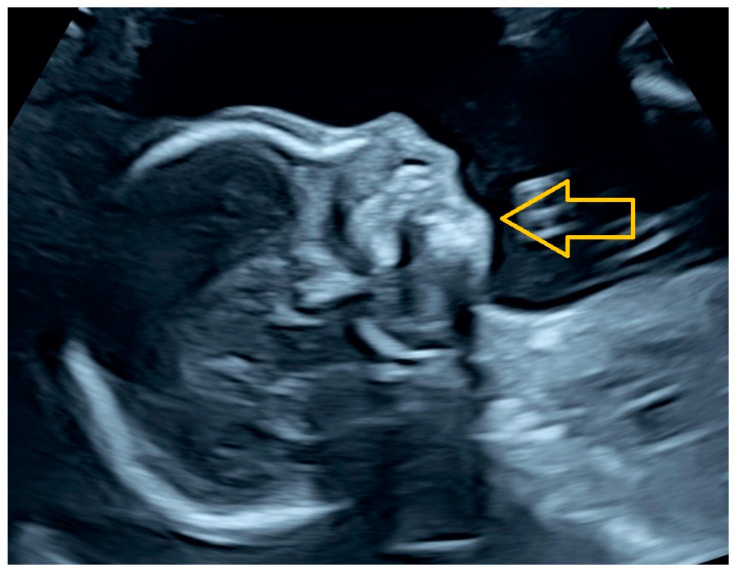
Results of a prenatal ultrasound: Marked micrognathia is evident in the sagittal fetal facial profile at 21 + 2 weeks of gestation, which is indicative of craniofacial involvement associated with monogenic connective tissue disorders. The arrow indicates the region of mandibular hypoplasia consistent with micrognathia.
